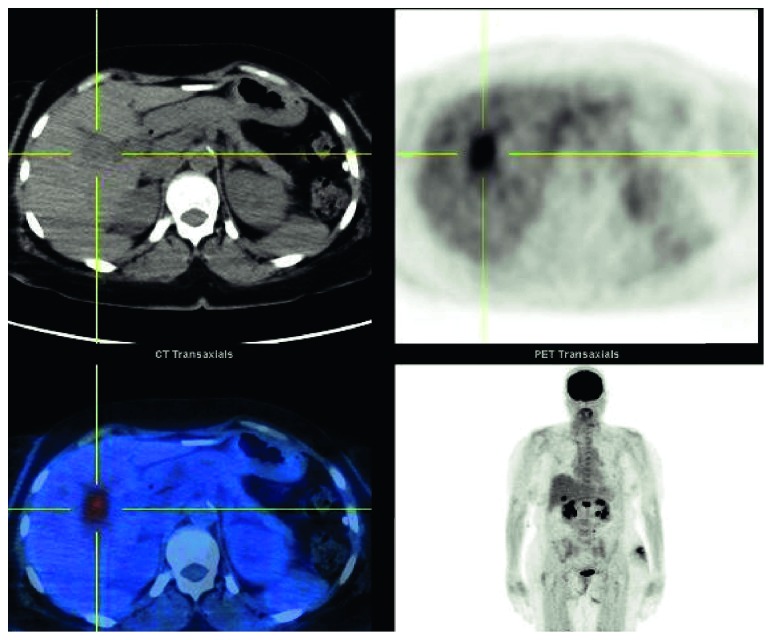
Follow-up PET after the 3^rd^ cycle of CHOEP. Interval resolution of supraclavicular, hilar, and axillary uptake. Increased uptake in the right hepatic lobe (SUV 5.8). New uptake in L1 (SUV 8.8). PET: positron emission tomography; CHOEP: cyclophosphamide, Adriamycin, vincristine, etoposide, and prednisone.
